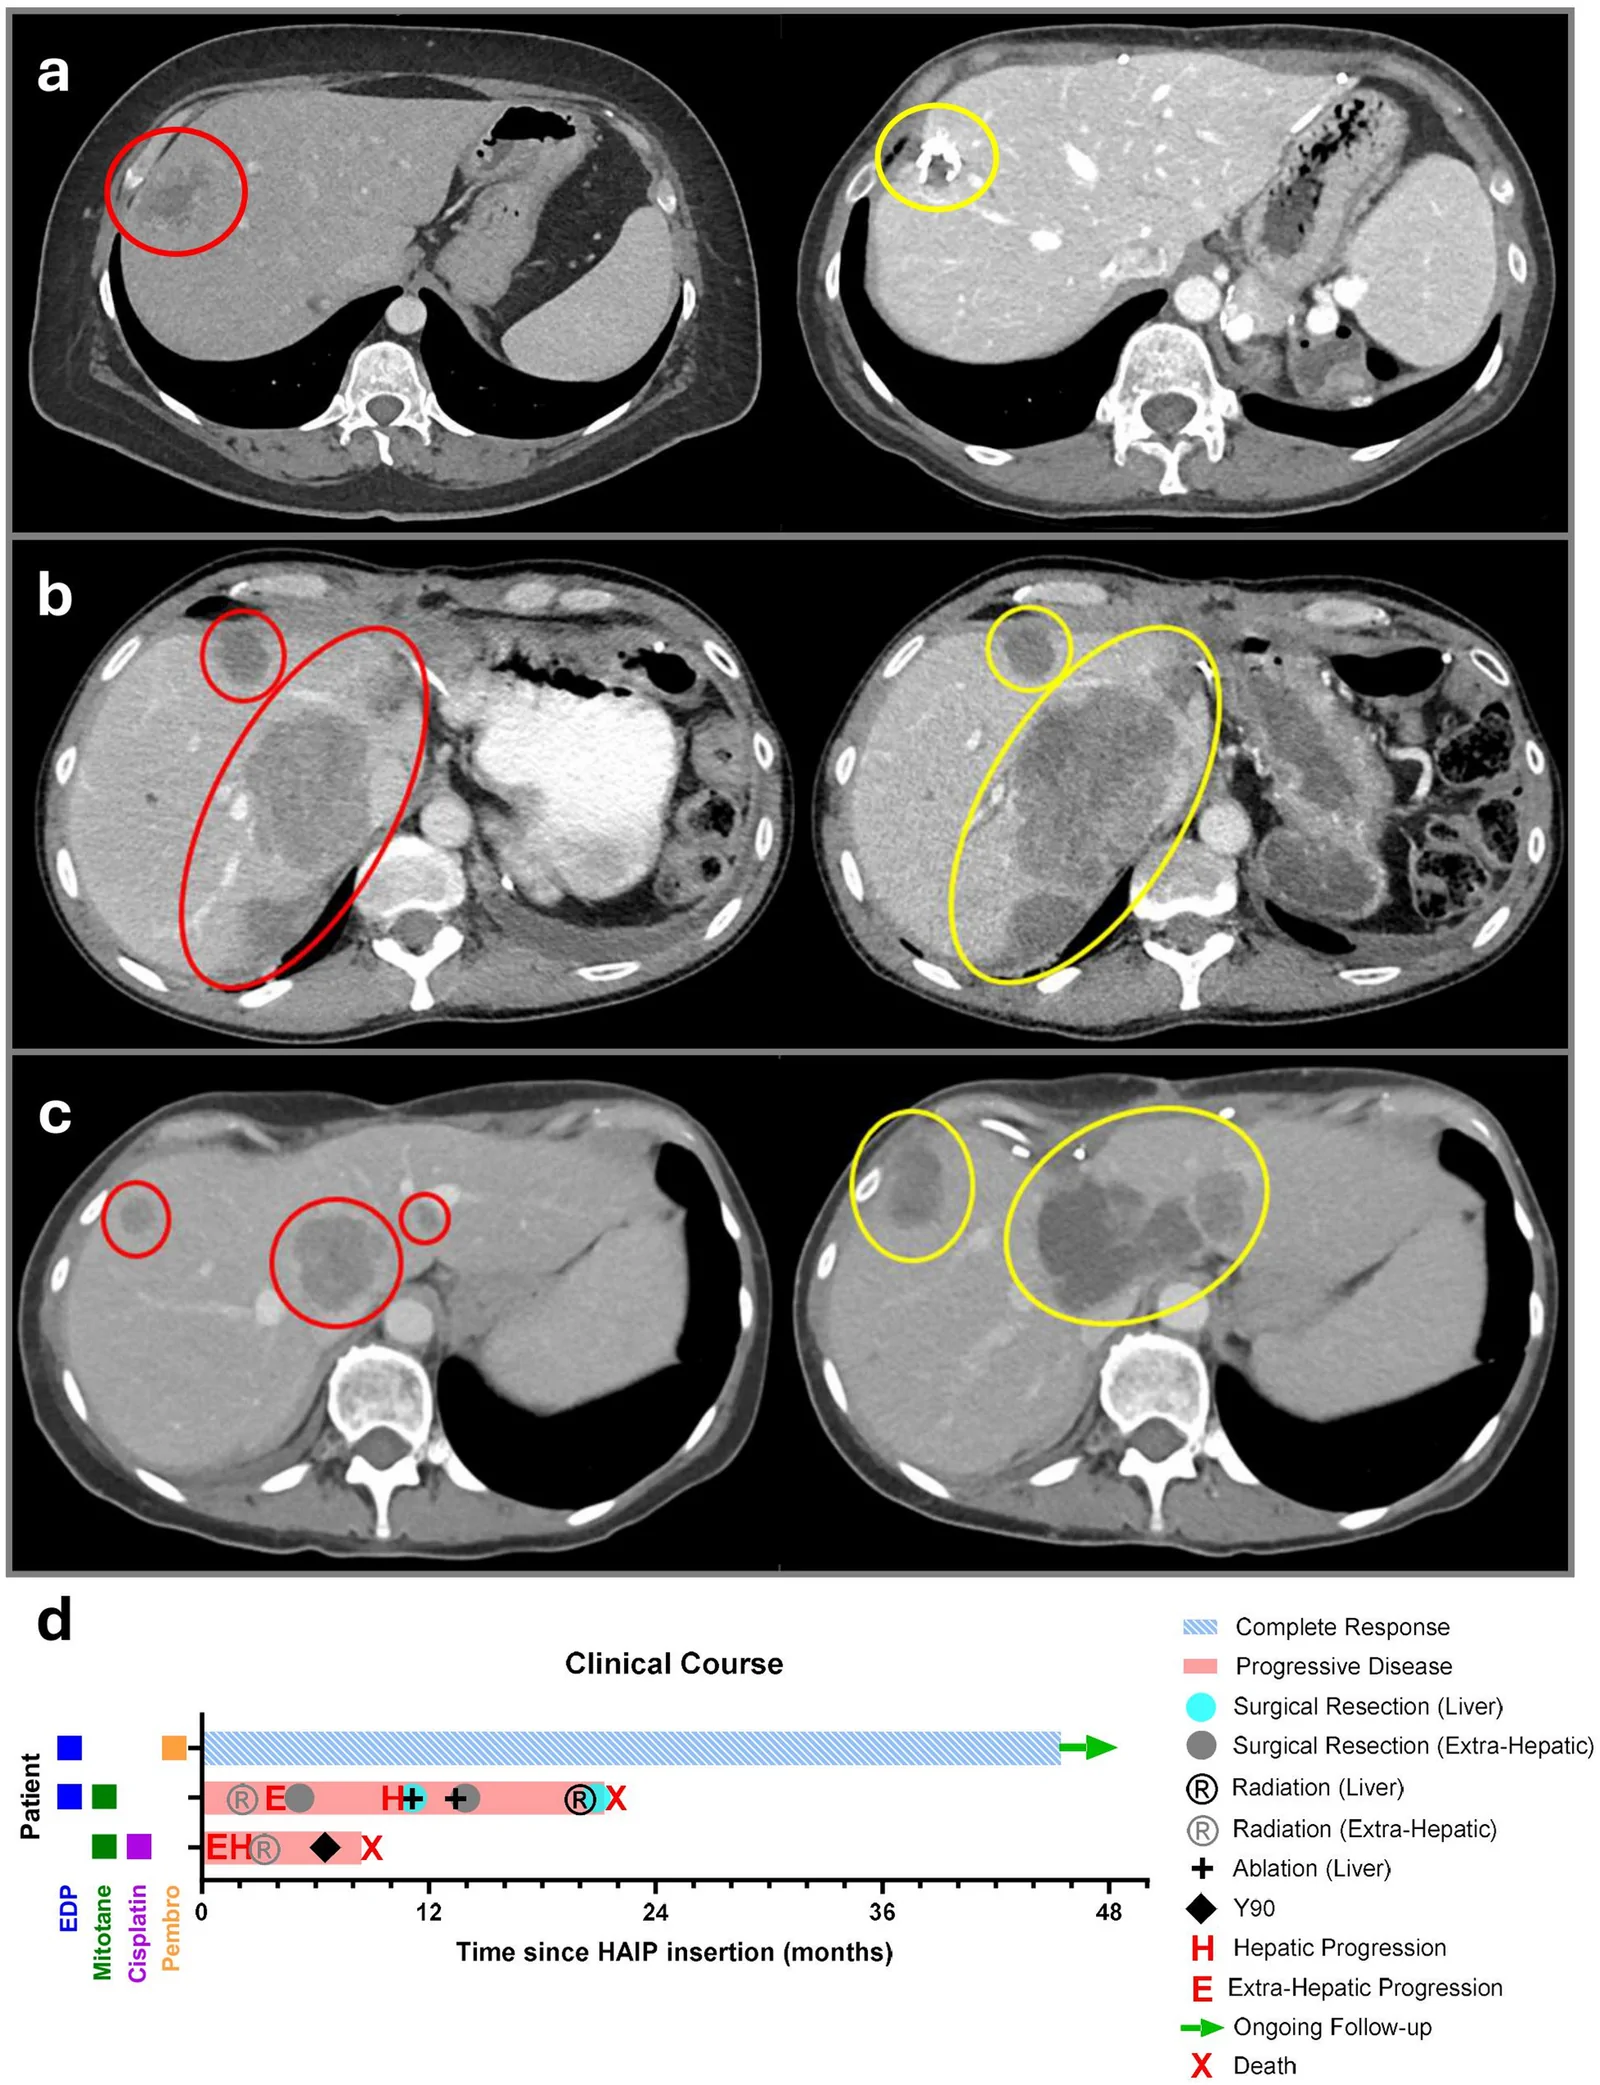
Patient Responses to HAIP-delivered Floxuridine for Locoregional Treatment ACC Liver Metastasis. (a) Axial CT comparing pretreatment heterogenous hepatic metastasis (red circle, left) compared to posttreatment expected post-surgical changes and clips within the liver (yellow circle, right) in patient with no evidence of recurrence at 44 months. (b) Axial CT demonstrating post-HAIP-treatment hepatic disease progression at 19 months (red circles, left) and 20 months (yellow circles, right) in a patient with progressive disease after transient control. (c) Axial CT comparing pretreatment hepatic disease (red circles, left) and post treatment hepatic disease progression at 2 months (yellow circles, right) in a patient with progressive disease. (d) Swimmer plots demonstrating patients’ clinical courses including disease response, progression, and both liver and extra-hepatic interventions. Top, middle, and bottom lines correlate with patient scans in (a), (b), and (c) respectively.
